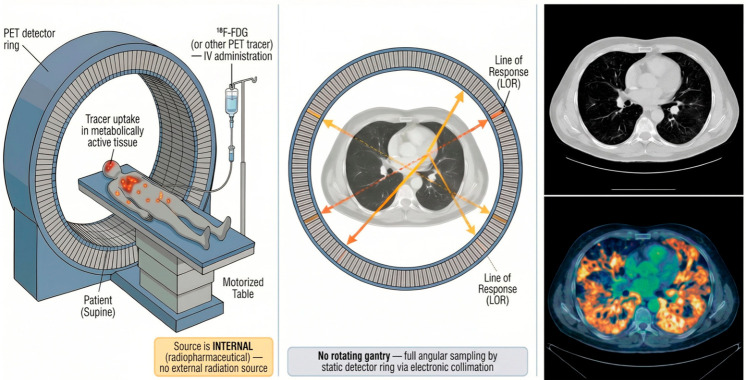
Instrumental setting (Left), response acquisition geometrical details (Center), and final rendering of computed tomography (CT) scan (Upper Right) and positron emission tomography (PET) scan (Lower Right). (Left): the intravenous contrast is metabolically active and is distributed into target tissues before the acquisition; therefore, the source of radiation is internal to the patient; (Center): the emission of divergent photons is sampled by a static detector ring. (Lower Right): PET image reconstruction with inflammation pattern, seen as different colors.
